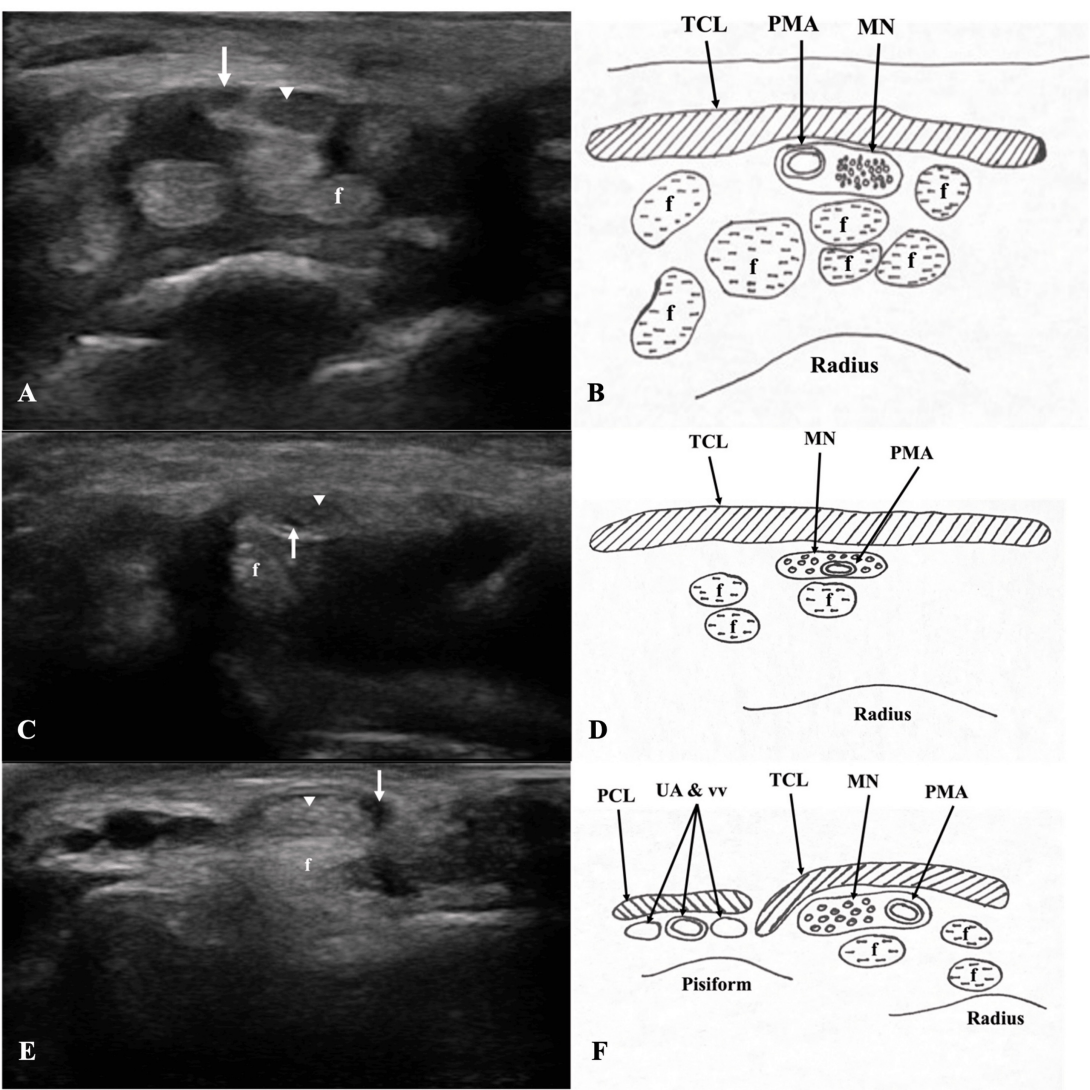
Ultrasonographic view of the persistent median artery in living subjects Transverse ultrasonographic images (A, C, E) and corresponding tracings (B, D, F) of the palmar PMA (white arrows) seen alongside the median nerve (white arrowheads) in the left carpal tunnel of three living subjects. Palmar PMA was found on the ulnar, dorsal, and radial sides of the median nerve in panels A, C, and E, respectively. f: flexor tendons; MN: median nerve; PCL: palmar carpal ligament; PMA: palmar persistent median artery; TCL: transverse carpal ligament; UA and vv: ulnar artery and veins
